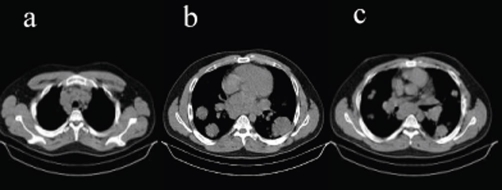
Non contrast chest CT scan, mediastinal window: multiple, bilateral, heterogeneous masses, with lobulated margins and mediastinal lymphadenopathy are noted.
